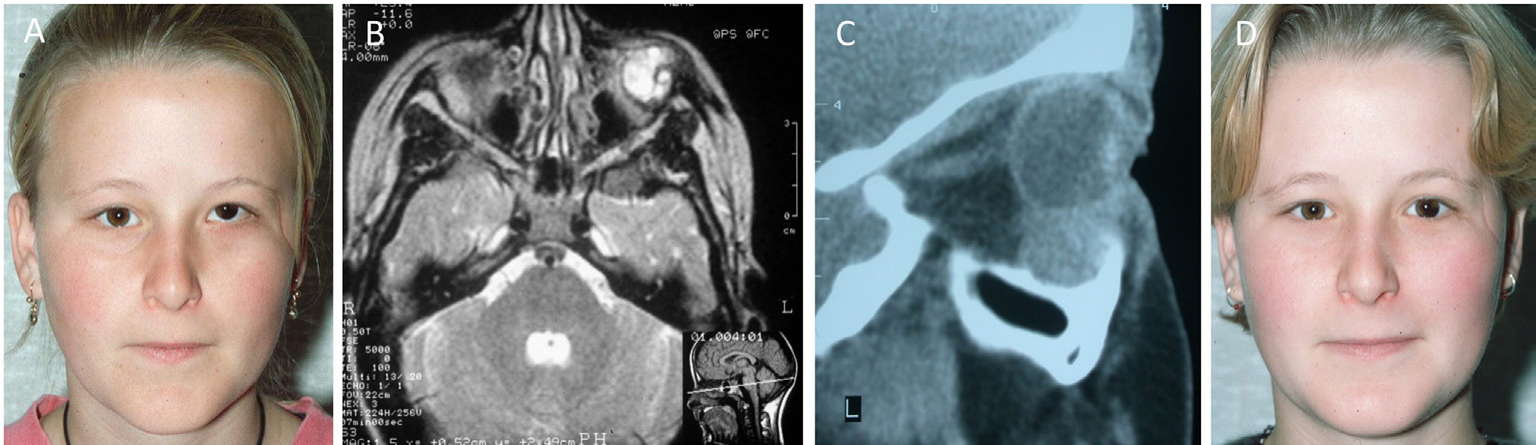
A 17-year-old female with a left orbital venous malformation causing ocular dystopia. The visible temporal scar resulted from a previous failed attempt of excision elsewhere. (A) A n MRI (B) and a CT scan (C) showed a 2 cm × 2 cm soft tissue mass on the floor of the left orbit displacing the globe superiorly and a bony ridge at the inferior orbital margin (C). The lesion was excised through a subciliary incision with removal of the bony lip and reduction of the hypertrophic orbital floor, restoring the position of the globe. Photograph 2 years postoperatively (D). Reproduced with permission from the British Journal of Oral and Maxillofacial Surgery (5).
